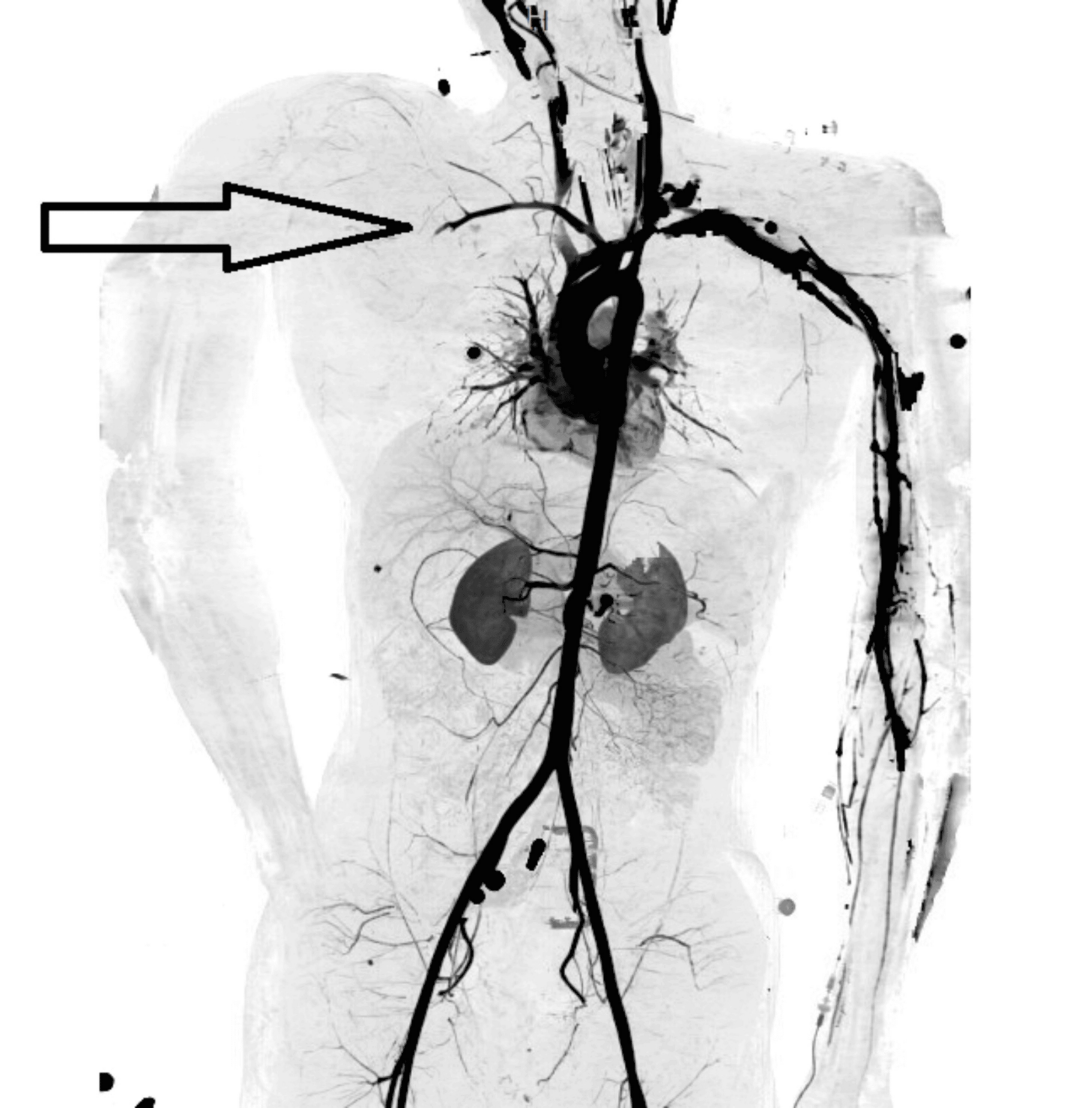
CT 3-D reconstruction view demonstrating no blood supply in the right upper limb The black arrow points to the lack of blood supply in the right upper limb blood vessels compared to the left upper limb.
